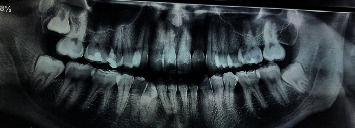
Panoramic radiograph from 2016 demonstrating a well-defined unilocular radiolucent lesion in the left mandible.
